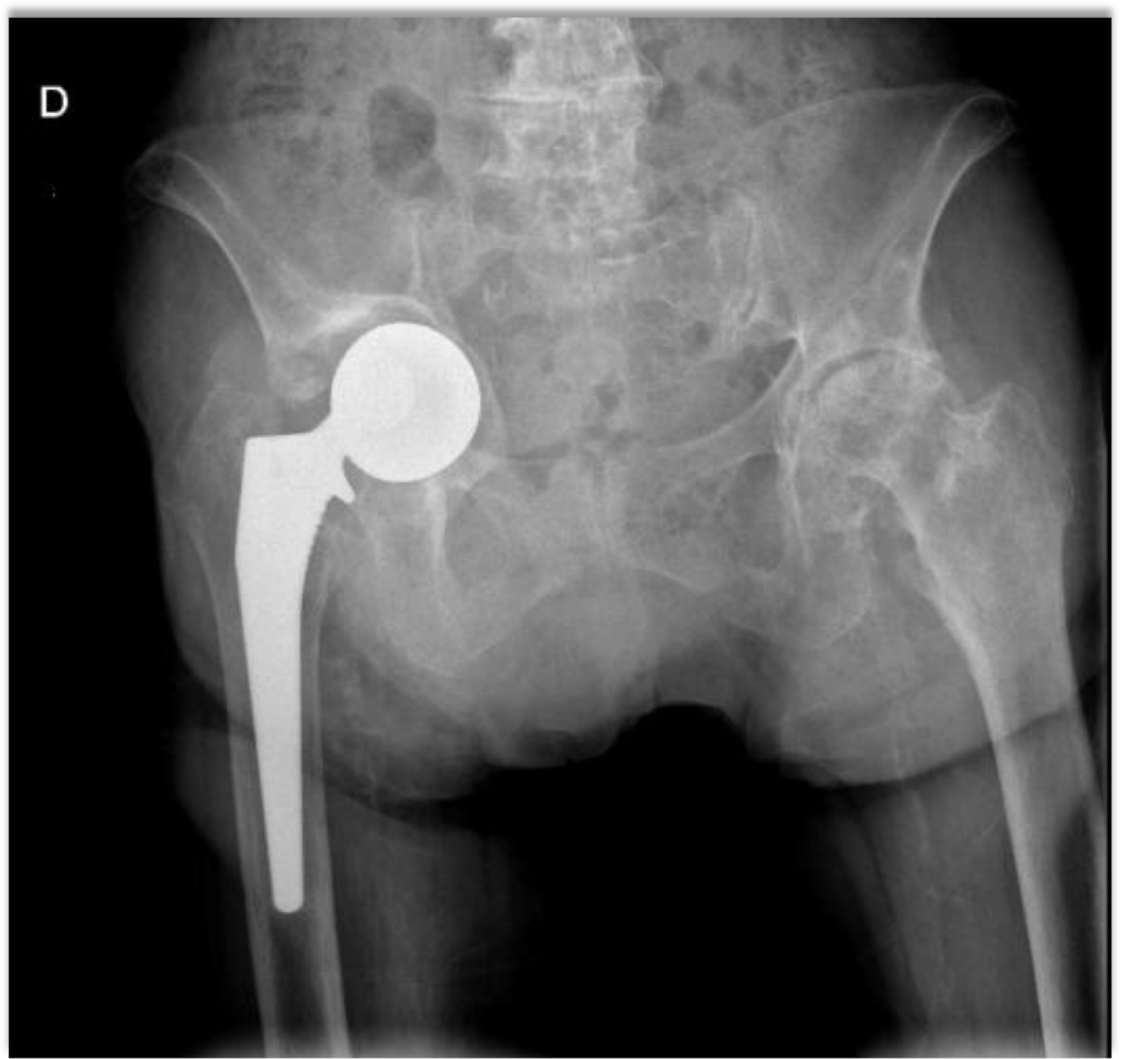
X-ray of a first generation Sunfit^®^ loosening case with secondary displacement.
